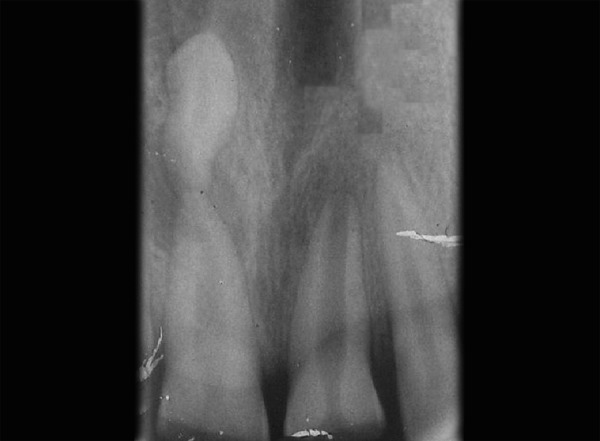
Periapical radiograph revealing inverted mesiodens apical to 11
